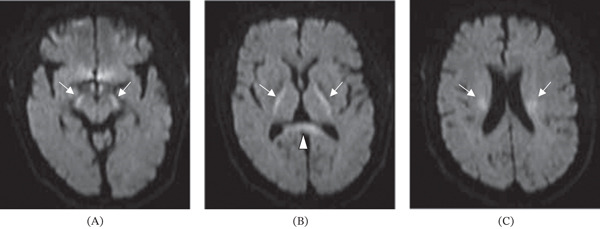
Magnetic resonance imaging of hypoglycemic encephalopathy. Axial diffusion‐weighted imaging performed 18 h after admission depicts hyperintensities of (A, arrows) bilateral cerebral peduncle, (B, arrowhead) splenium of corpus callosum, (B, arrows) posterior limb of internal capsule, and (C, arrows) corona radiata.
